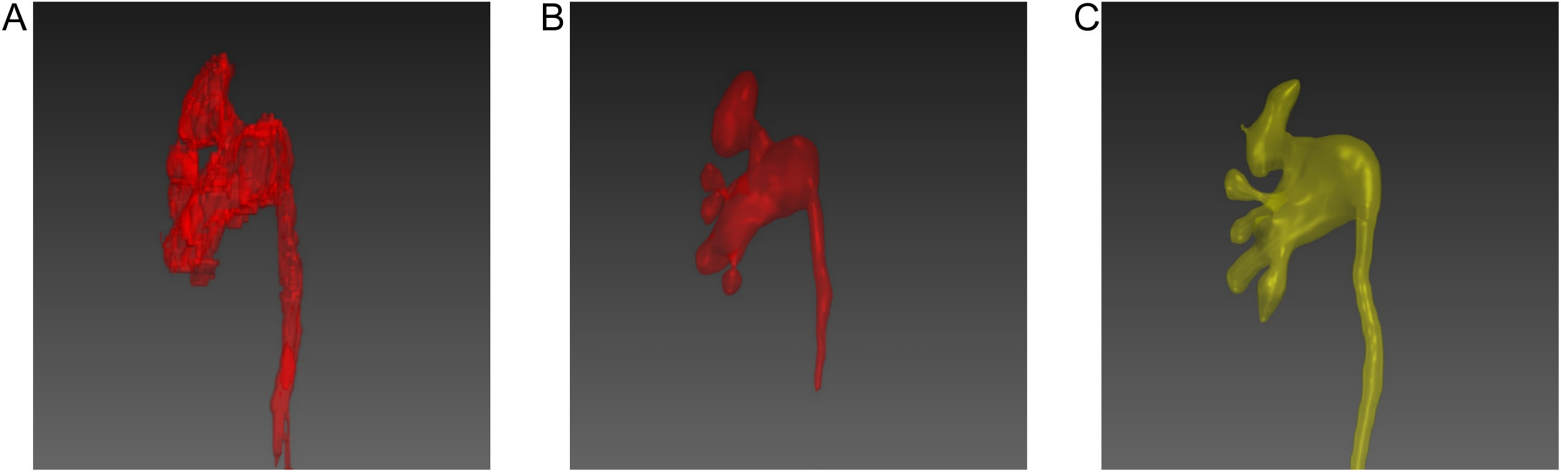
Anterior view of distended PCS being 3D reconstructed based on excretory and native phases. A, reconstruction of the non-contrast 3D model before smoothing; B, reconstruction of the non-contrast 3D model after smoothing; C, 3D reconstruction based on CT images of excretory phase. PCS, pelvicalyceal system, 3D, 3 dimensional; CT, computed tomography.
